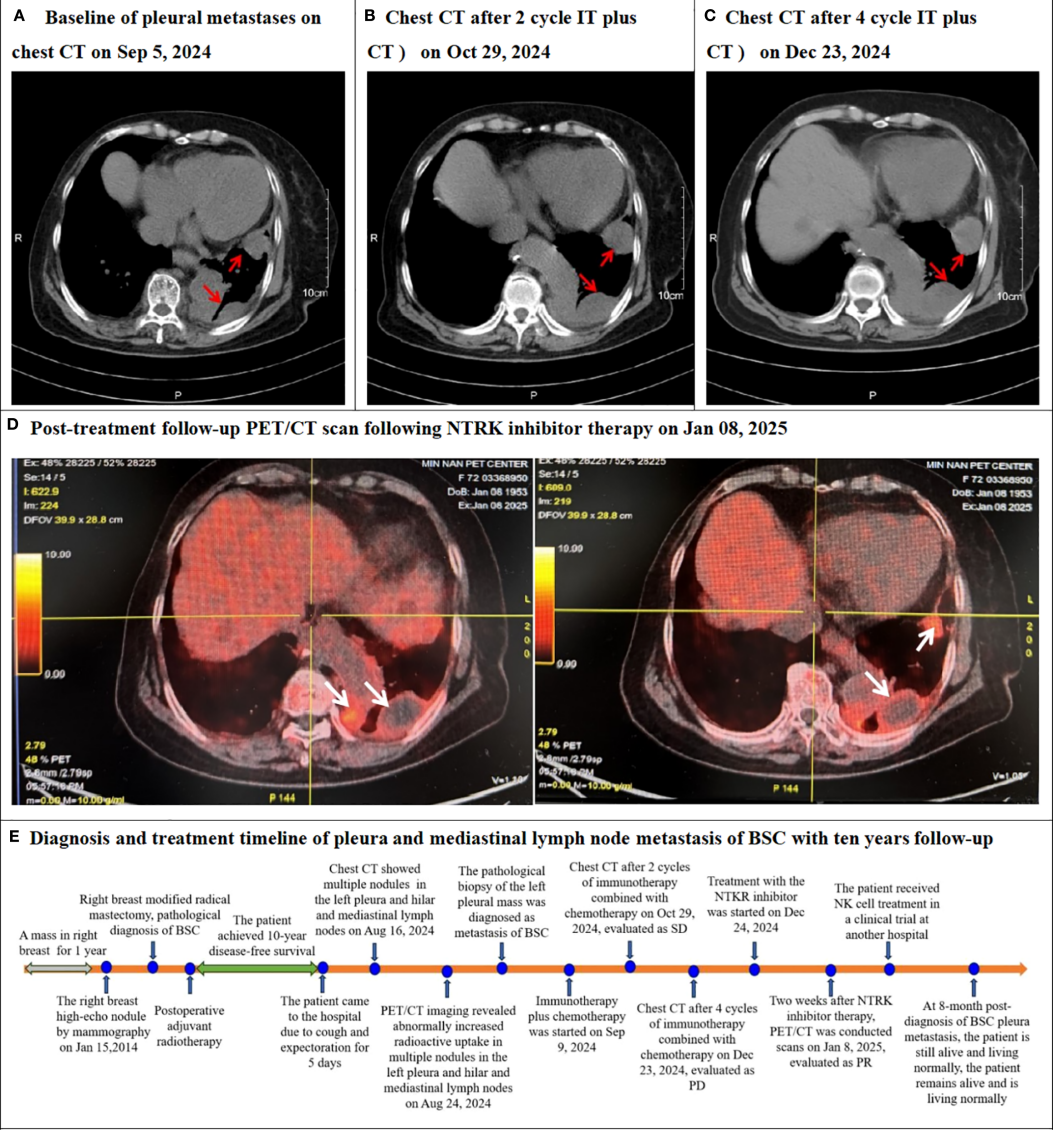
Chest CT or PET/CT after re-examination following systemic therapy and timeline of this case. (A) The Baseline chest CT scan performed on Sep 5, 2024, revealed left pleura masses (red arrow). (B) The follow-up chest CT after systemic treatment (2 cycles of immunotherapy combined with chemotherapy) on Oct 29, 2024, evaluated as stable disease (SD). (C) The follow-up chest CT after systemic treatment (4 cycles of immunotherapy combined with chemotherapy) on Dec 23, 2024, evaluated as progressive disease (PD). (D) Post-treatment follow-up the whole body positron emission tomography/computed tomography (PET/CT) scans on Jan 8, 2025, following NTRK inhibitor therapy, demonstrated reduced tumor mass and significantly diminished hypermetabolic activity (white arrows), consistent with partial response (PR). (E) Diagnosis and treatment timeline of pleura and mediastinal lymph node metastasis of BSC with ten years follow-up.
